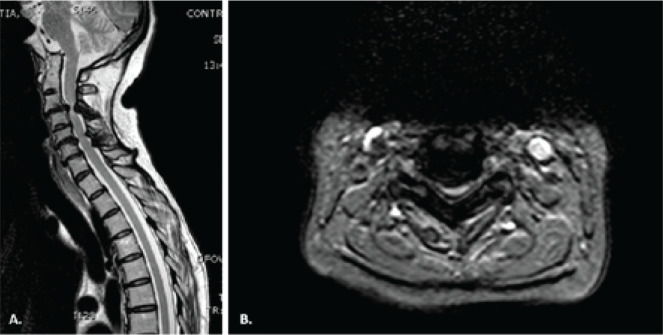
MRI of the neck. Subaxial subluxation (A - sagittal plane) and spinal compression at the C3 level (B - axial plane).
